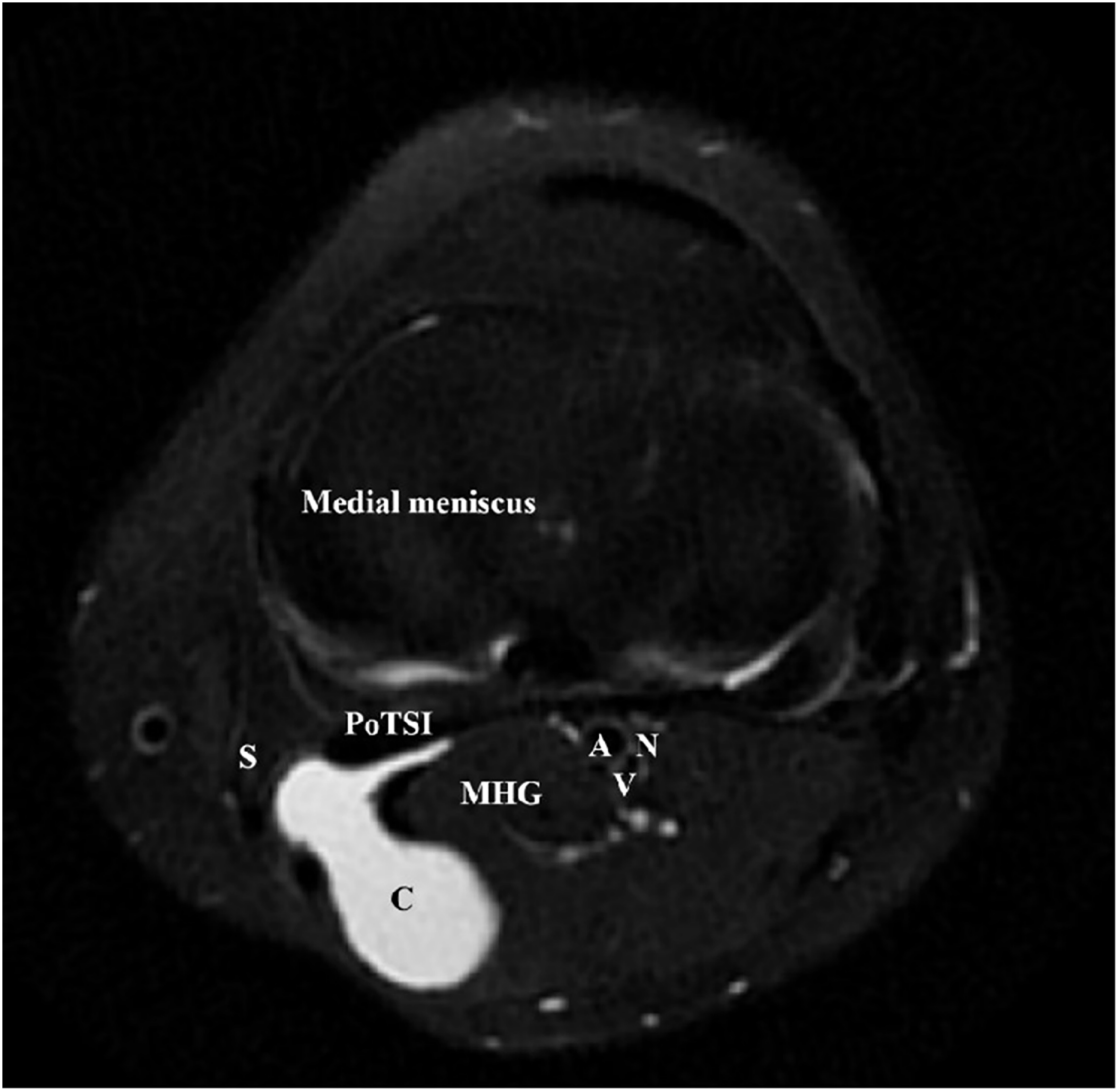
Axial MRI of a left knee demonstrating a popliteal cyst. Popliteal cyst (C) lied in between the gastrocnemius (MHG) and semimembranosus (S). The neurovascular bundle was protected by the medial head of gastrocnemius. The medial head of the gastrocnemius and semimembranosus were labeled for anatomical landmarks.
